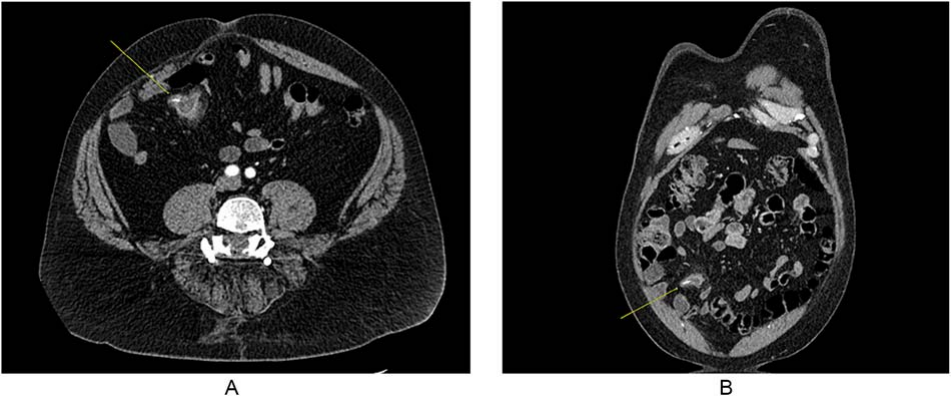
CT imaging showing foreign body in MD, with some inflammation and fat stranding around it. (A) Coronal imaging and (B) cross-sectional.
